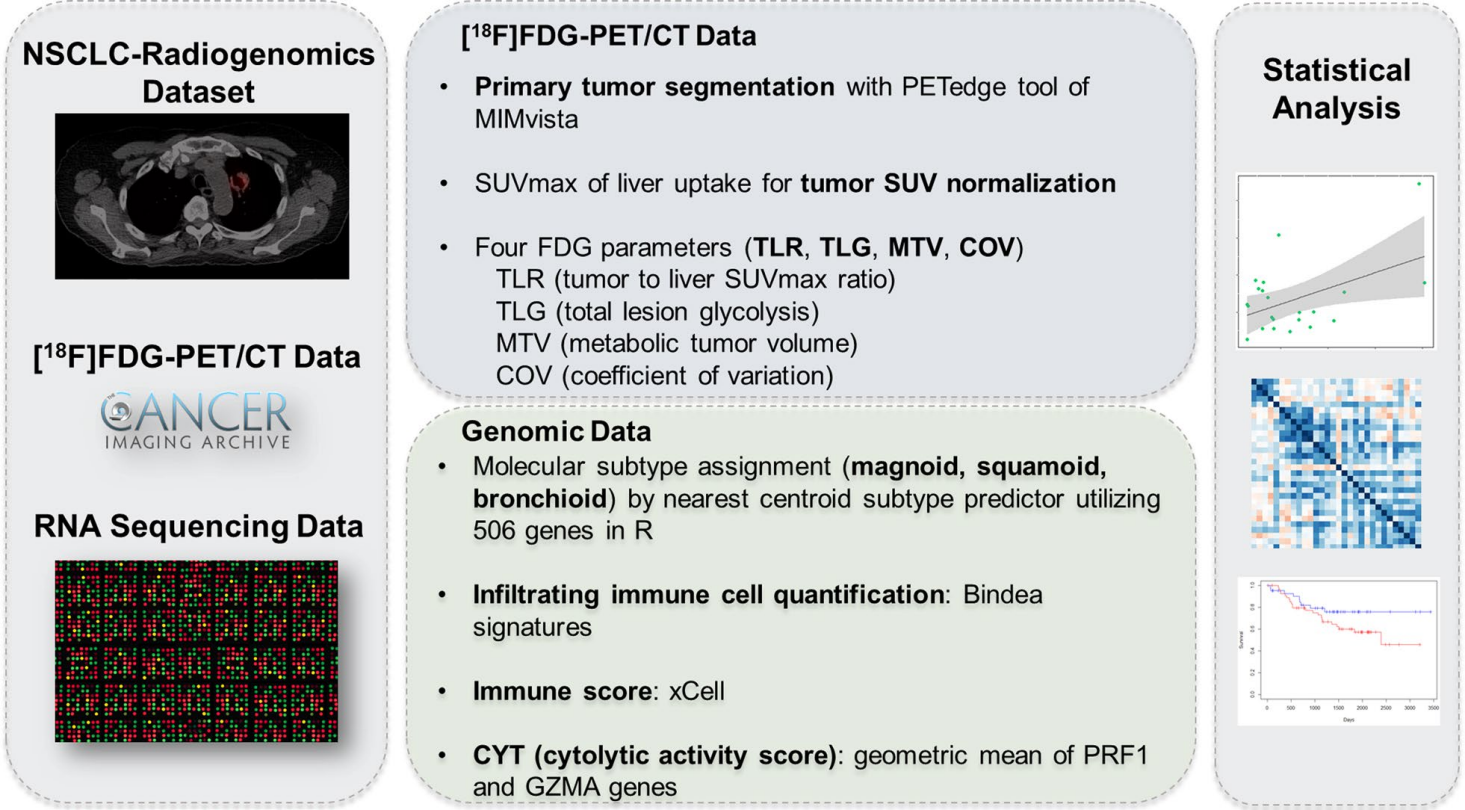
Scheme of the study. ^18^F-FDG PET and RNA sequencing data were obtained from NSCLC radiogenomics dataset in TCIA. Four ^18^F-FDG parameters were measured from manually assigned ROI of primary tumor. RNA sequencing data used for molecular subtype assignment and immune-related quantification. Statistical analysis was performed by integrating ^18^F-FDG parameters and genomic signatures
